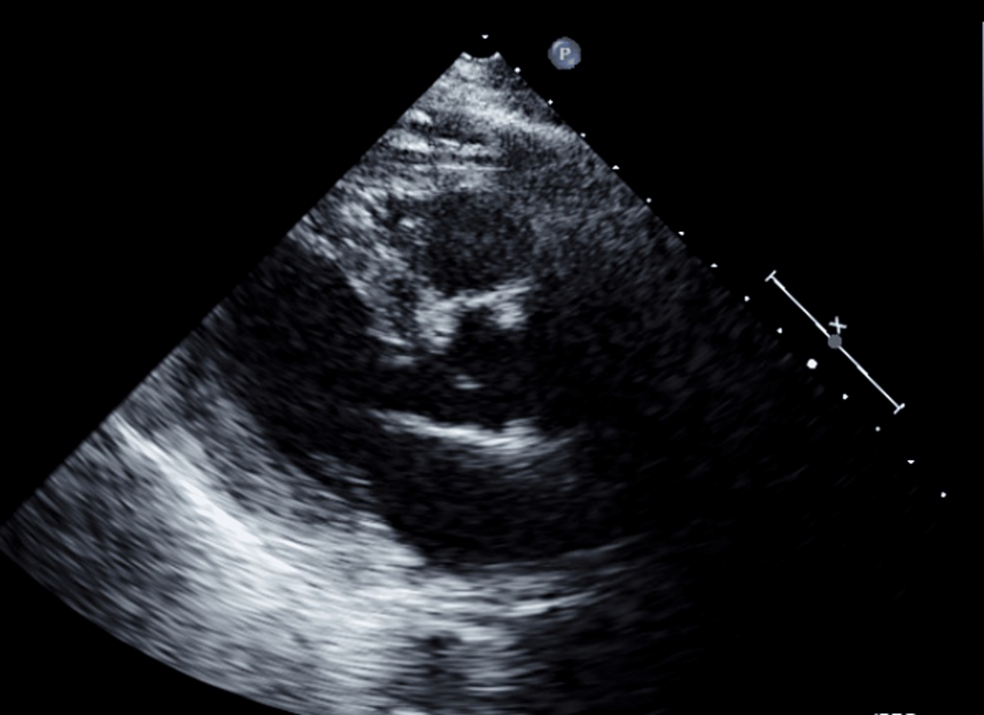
Still of echocardiography. The hematoma was not observable in the echocardiography view and was not compressing any cardiac chambers.
